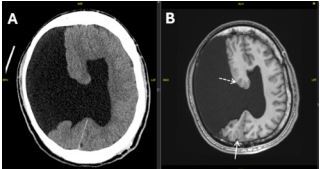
(A) Large right frontoparietal open-lip schizencephaly demonstrated on non-contrast computed tomography. (B) T1-weighted non-contrast magnetic resonance imaging demonstrates heterotopic gray matter (dashed arrow) and polymicrogyria (solid arrow).
